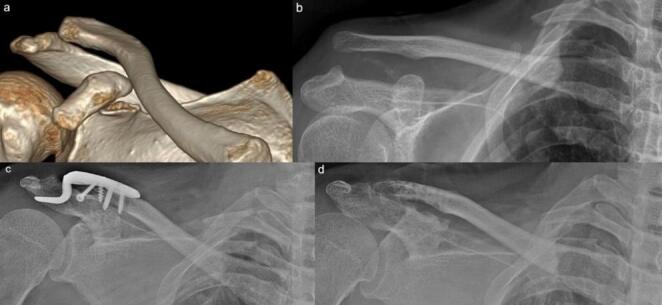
a Three-dimensional shoulder CT showing a displaced coracoid process fracture. b A Rockwood type V acromioclavicular (AC) joint injury was diagnosed at a delayed stage. c At 7 weeks post-injury, a modified Weaver-Dunn procedure was performed. d At the final follow-up, the AC joint was well reduced
